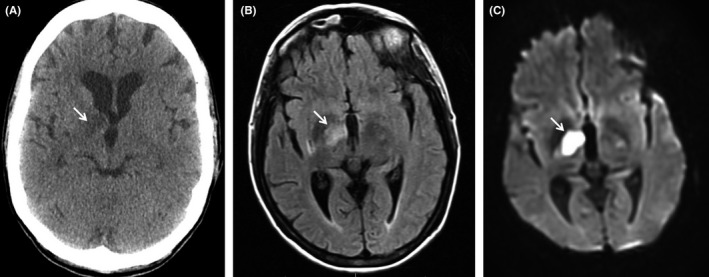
Radiologic studies of the brain. (A) Computed tomography scan of the brain showing 1.5‐cm lucency involving the right thalamus, which potentially suggests acute infarct as one of the differentials. (B) T1‐weighted magnetic resonance imaging that shows increased focal density involving right thalamic area. (C) Diffusion‐weighted magnetic resonance imaging which demonstrates an area of acute infarct in the right thalamus measuring approximately 2.1 × 1.3 cm^2^ in the greatest dimension
